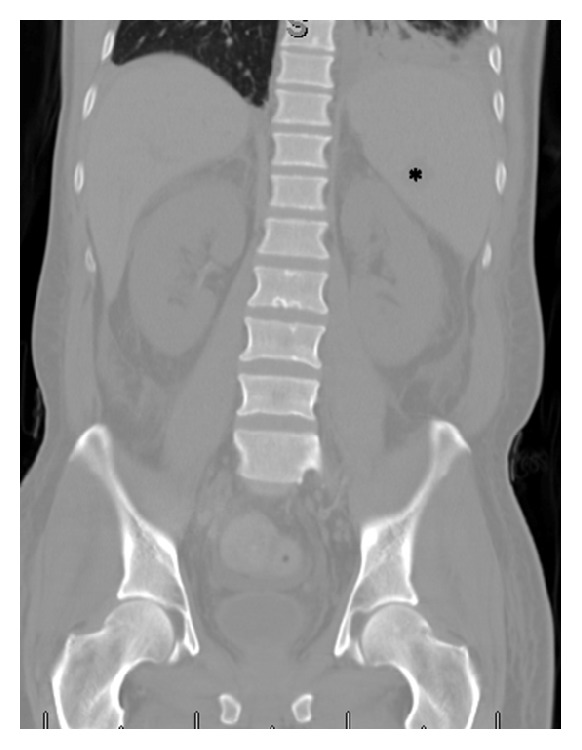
Coronal reconstruction CT in bone window at the time of admission to the outside institution shows essentially normal bone marrow other than some Schmorl's nodes. Note additional findings of splenomegaly and left basilar consolidation/atelectasis. Focus of hypoattenuation in the spleen (*∗*) was interpreted as a focus of splenic infarction. Also note that there is no evidence of osteonecrosis in either hip.
